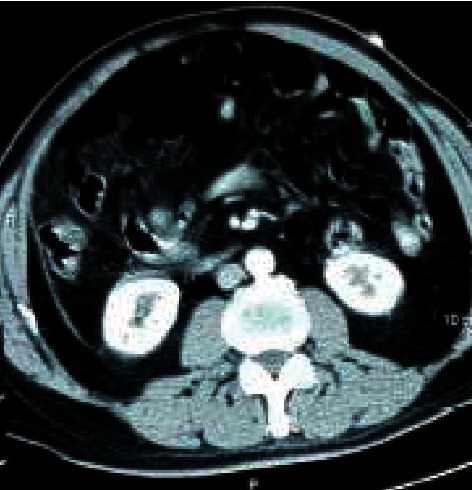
Abdominal CT scan: nonconclusive finding.
